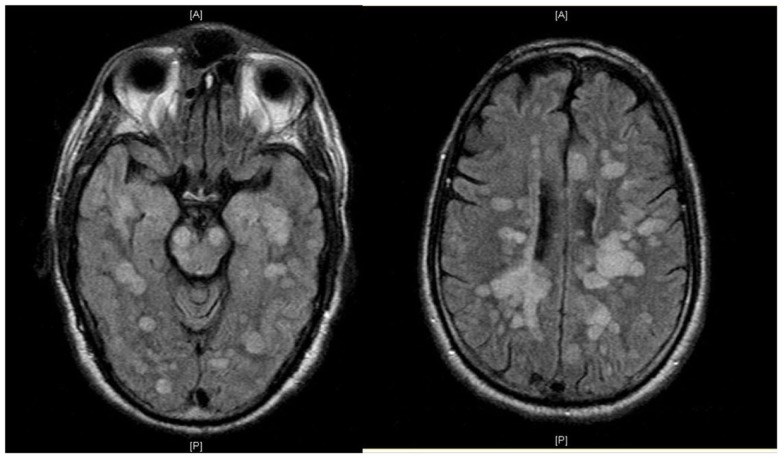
MRI (FLAIR) shows increased number of supra and infratentorial lesions.
